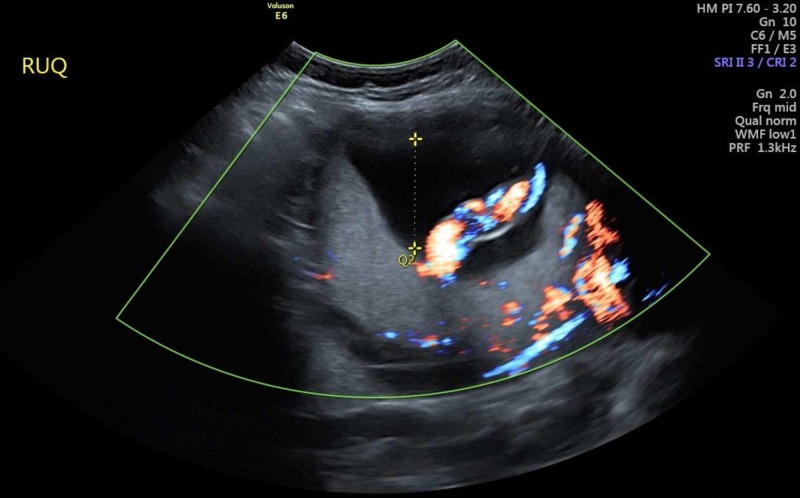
Ultrasound evaluation of the patient demonstrating a lack of evidence of PASD at the fundus
